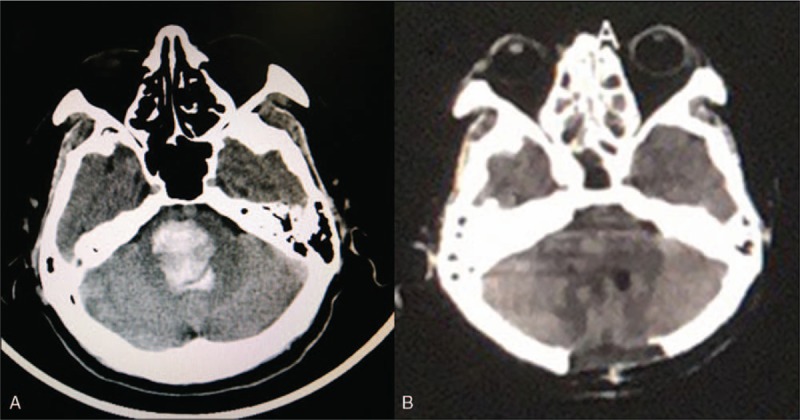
Preoperative CT scan showed a large hematoma mainly located on pontine extending to fourth ventricle (A); Postoperative CT showed mostly removal of the hematoma (B).
